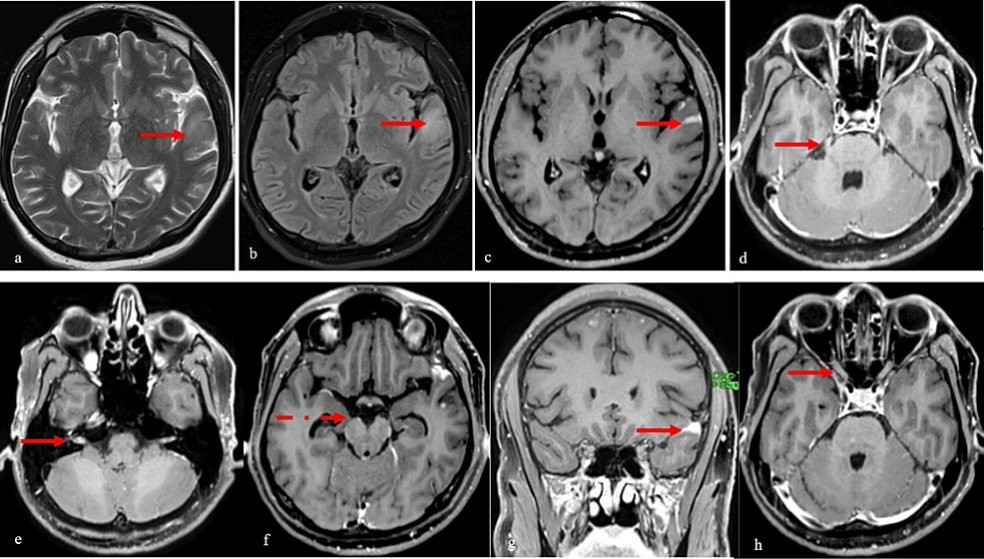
MRI of the brain Axial T2WI (a) demonstrates a discrete focus of hyperintensity with the corresponding hyperintensity on axial FLAIR (b) and non-enhancement of the intraparenchymal focus with vivid nodular gyriform enhancement of the overlying gyri of axial post-contrast T1WI (c). Nodular perineural enhancement of the cisternal segments of bilateral trigeminal (arrow in d), meatal segments of the bilateral facial (arrow in e), the pial surface of the right oculomotor nerve (dashed arrow in f), and prechiasmatic and intracanalicular segments of bilateral optic nerves (arrow in h) are identified on multi-level axial sections of post-contrast T1WI (d,e,f, and h, respectively). Multiple foci of punctate and gyriform nodular enhancement are identified in bilateral cerebral hemispheres on coronal post-contrast T1WI (g). T1WI: T1 weighted image, T2WI: T2 weighted image, FLAIR: Fluid-attenuated inversion recovery
